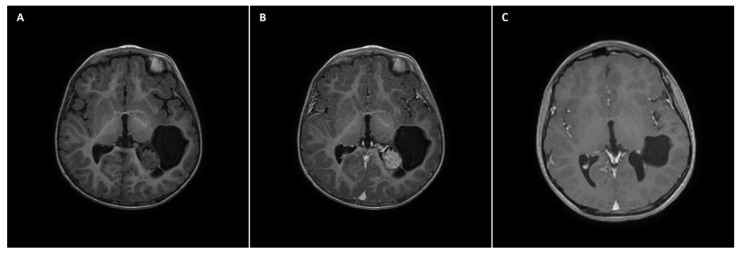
A 21-month-old boy with choroid plexus papilloma of the left lateral ventricle. (A) A preoperative axial non-contrast T1 MRI scan with a hypodense mass located within the left occipital horn of the lateral ventricle with cystic components. (B) The preoperative axial postcontrast T1 MRI scan showed homogeneous intraventricular mass enhancement, reflective of choroid plexus papilloma. (C) A postoperative axial postcontrast T1 MRI scan reflecting complete excision of the intraventricular mass, with the remaining cystic component.
